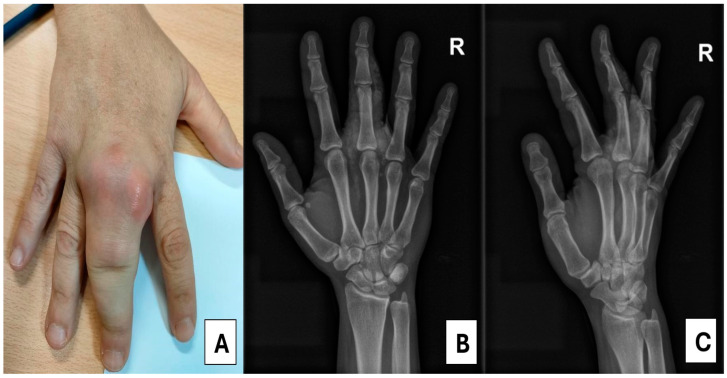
(A) Right hand showing a lobulated, cystic-appearing mass; (B,C) X-rays of the right hand revealing calcifications localized in the proximal phalanx of the third finger.
